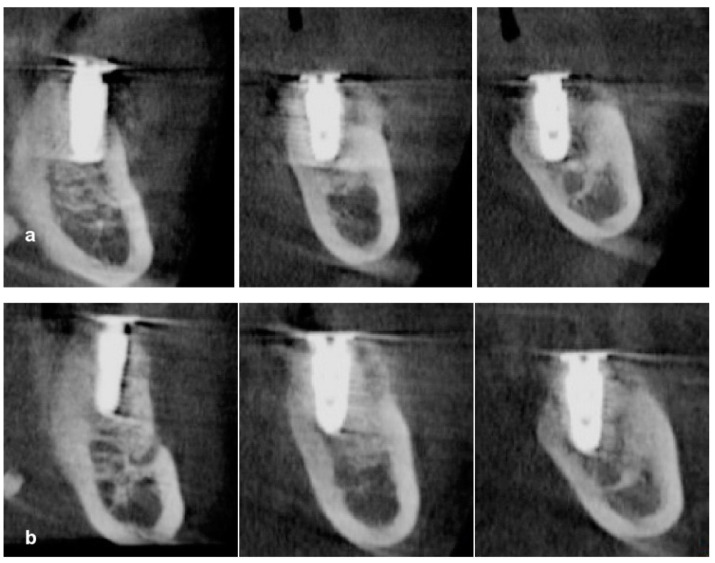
(a) CBCT scans immediately after surgery. (b) CBCT scans after 5 months of healing reveal successful ridge augmentation on bony defect and over implant platforms.
